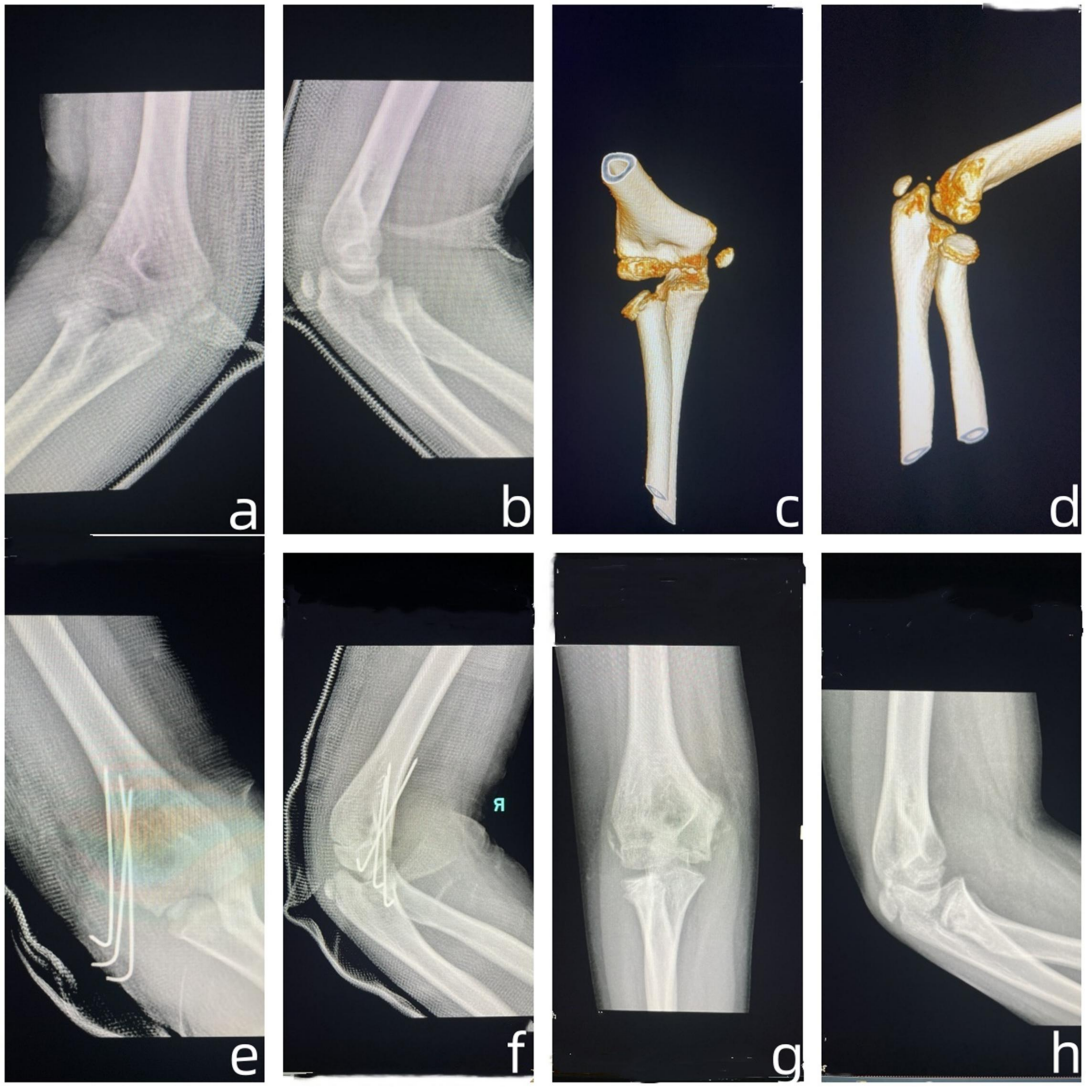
(a) One 10 years boy, fall from height, 1 day after injury the Anteroposterior radiographs; (b) 1 day after injury the Lateral radiographs; (c) 2 day after injury the Anteroposterior Three dimensional CT; (d) 2 day after injury the Lateral Three dimensional CT; (e) 1 day post operation the Anteroposterior radiographs; (f) 1 day post operation the Lateral radiographs; (g) 3 months post operation the Anteroposterior radiographs; (h) 3 months post operation the Lateral radiographs.
